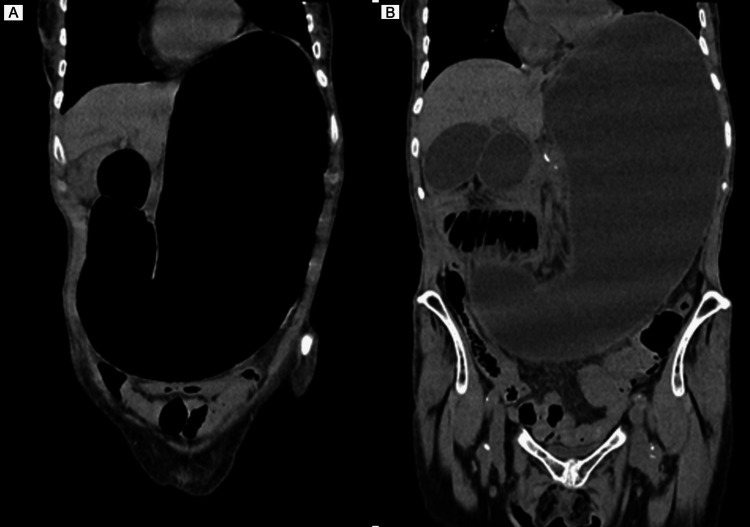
(A, B) Coronal view of abdominal CT showing gastroduodenal distention of the stomach until the third part of the duodenum. CT: computed tomography
